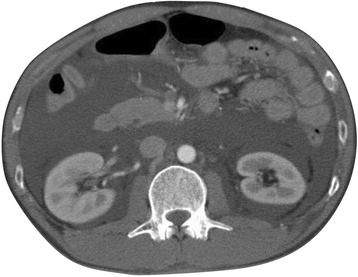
Postoperative abdominal CT revealed fluid accumulation in the peritoneal cavity and retroperitoneum
